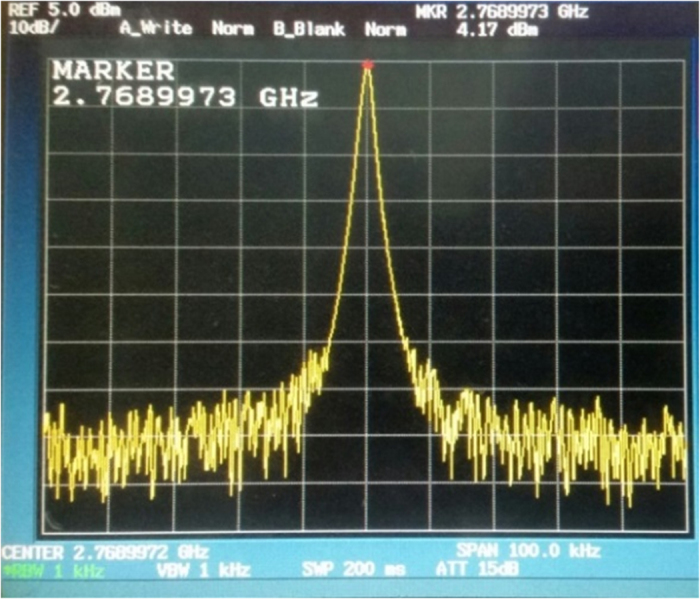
Natural frequency. Before applying a Doppler-shifted signal to the oscillator, the spectrum of the natural frequency was measured at 2.76 GHz.
